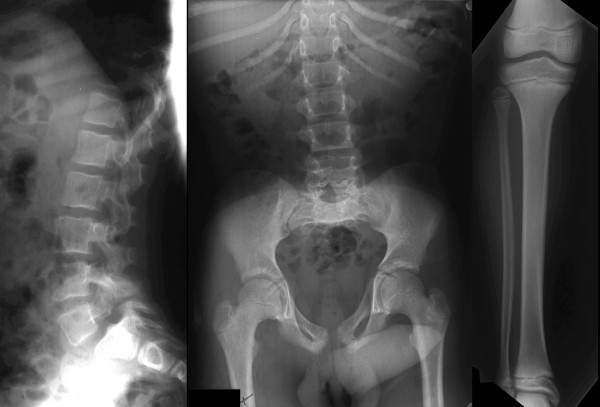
Radiographic features of the adult 3-M syndrome patient. On the lateral lumbar spinal radiograph (left panel), vertebral bodies are relatively tall and short. Lumbar hyperlordosis is caused by the short femoral necks. In the central panel, note the short femoral necks with anterior rotation of the pelvis (small foramina obturatoria). The ribs and the visible part of the femoral shafts are slender. The distance between the vertebral pedicles remains constant from L1 to L5. The tibia and fibula (right panel) are relatively inconspicuous. The radiographic features fitting with the diagnosis of 3-M are the tall and narrow vertebral bodies and slender tubular bones; other features of a chondrodysplasia, such as platyspondily or metaphyseal and epiphyseal changes, are typically absent.
